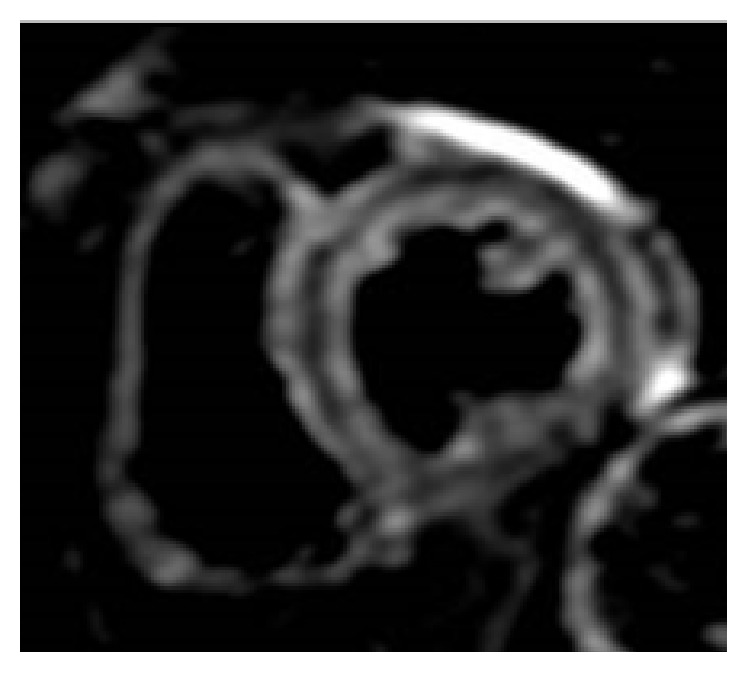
T2-weighted sequences cardiac MRI of the patient. There is a bright white rim of enhancement lining the lateral wall of left ventricle, which is consistent with subendocardial edema.
